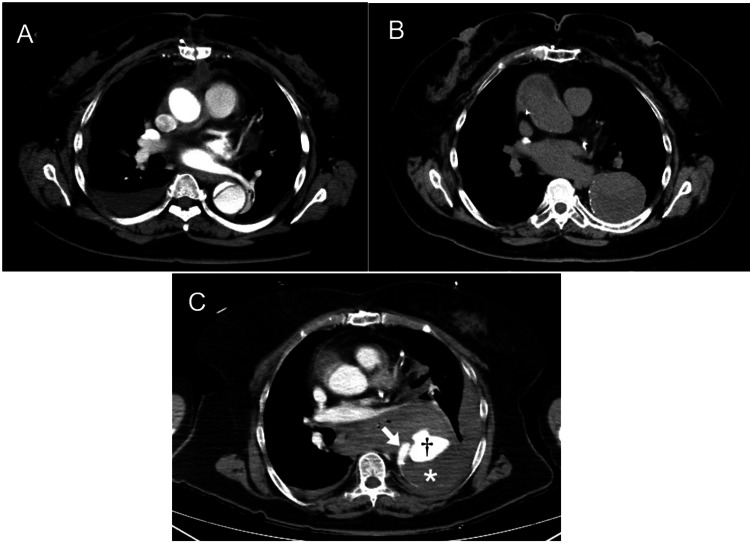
A: CT scan showed a residual dissection after the initial operation. B: Follow-up CT scan showed an increasing diameter of the descending thoracic aorta. C: Contrast-enhanced CT scan revealed rupture (†) of the newly formed false lumen in the triple-barreled dissection. The arrow indicates the true lumen; the asterisk (*) indicates the previous false lumen; and the dagger (†) indicates the newly formed false lumen.
